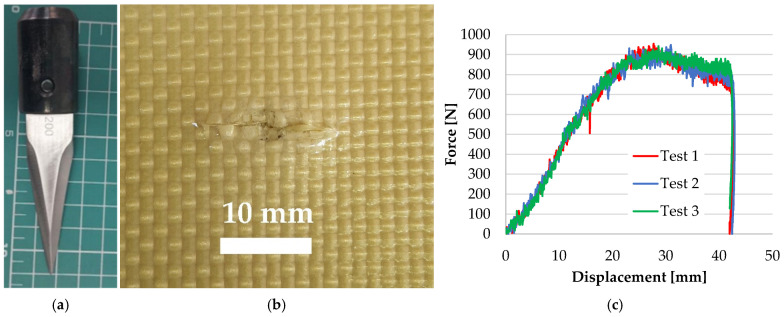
(a) Knife S1, with the blade geometry as in [80]; (b) the face of first layer from a panel of 16 layers of Twaron^®^ CT 736 CMP (thickness of 8.44 mm), after a test with a strike energy of 24 J (test carried out on INSTRON CEAST 9350 drop tower impact system, at INCAS Bucharest, Romania) [67]; (c) force–displacement curves for the three tests for the 16-layer panel of Twaron^®^ CT 736 CMP, under the same conditions (knife S1, 24 J).
